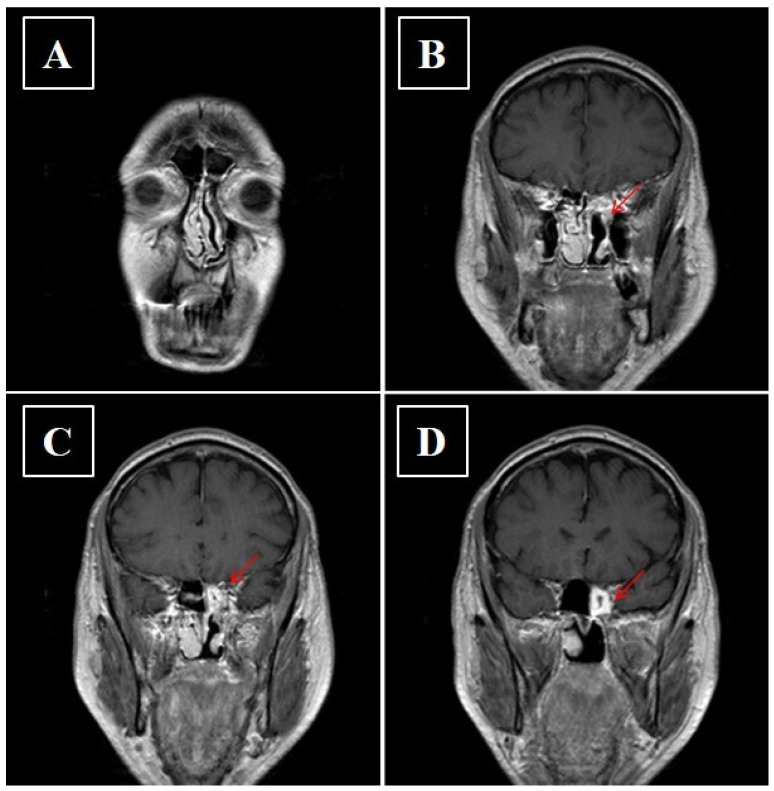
(A–D) Axial contrast enhanced fat-suppressed T1-weighted image showing reduction of the pathological tissue component in the upper offshoot of the ethmoid after adjuvant radiotherapy.
